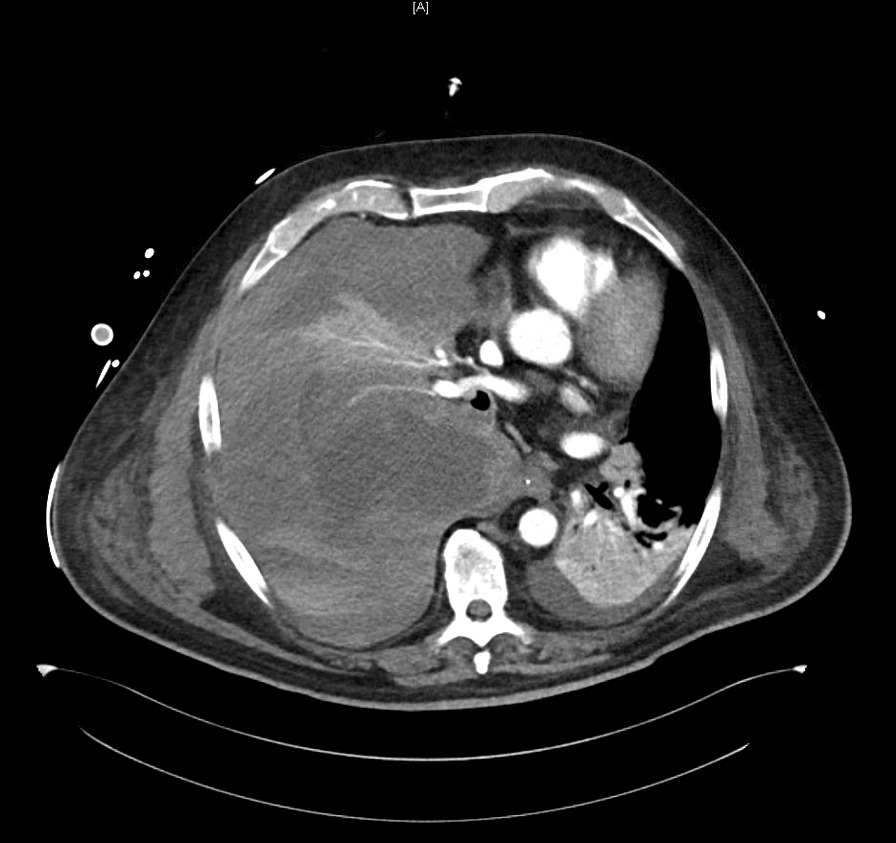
Axial slice from arterial phase computed tomography (Se:8, lm:59)
